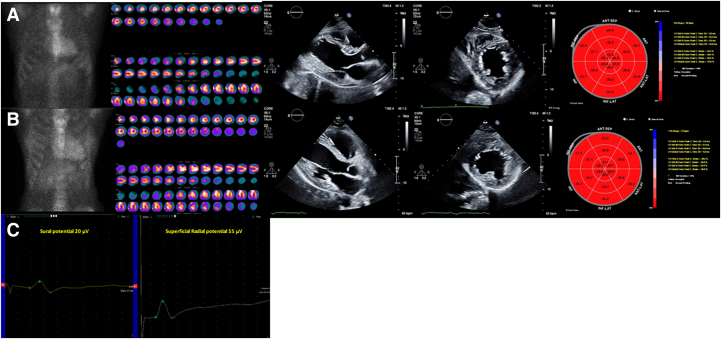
Cardiac Imaging and Nerve Conduction Findings in a Patient With TTR Trp61Leu Markedly abnormal planar (left) and cardiac (middle) single-photon emission computerized tomography images from technetium pyrophosphate studies despite normal echocardiographic findings (right) in (A) patient #3 (anteroseptal wall thickness 1.0 cm; inferolateral wall thickness 0.9 cm; global longitudinal strain −41.6%) and (B) patient #4 (anteroseptal wall thickness 0.9 cm; inferolateral wall thickness 0.9 cm; global longitudinal strain −36.6%). (C) Abnormal nerve conduction study tracings in patient #4.
